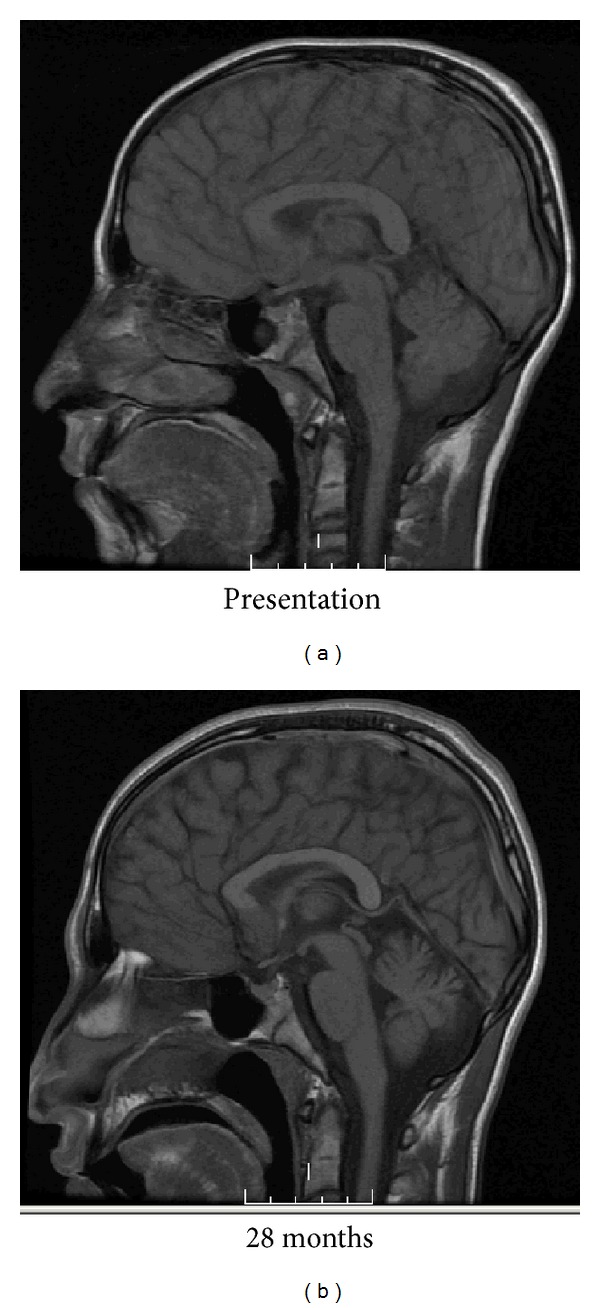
MRI demonstrates progressive atrophy of the corpus callosum, cortex, and cerebellum more than 2 years after the diagnosis of SSPE.
